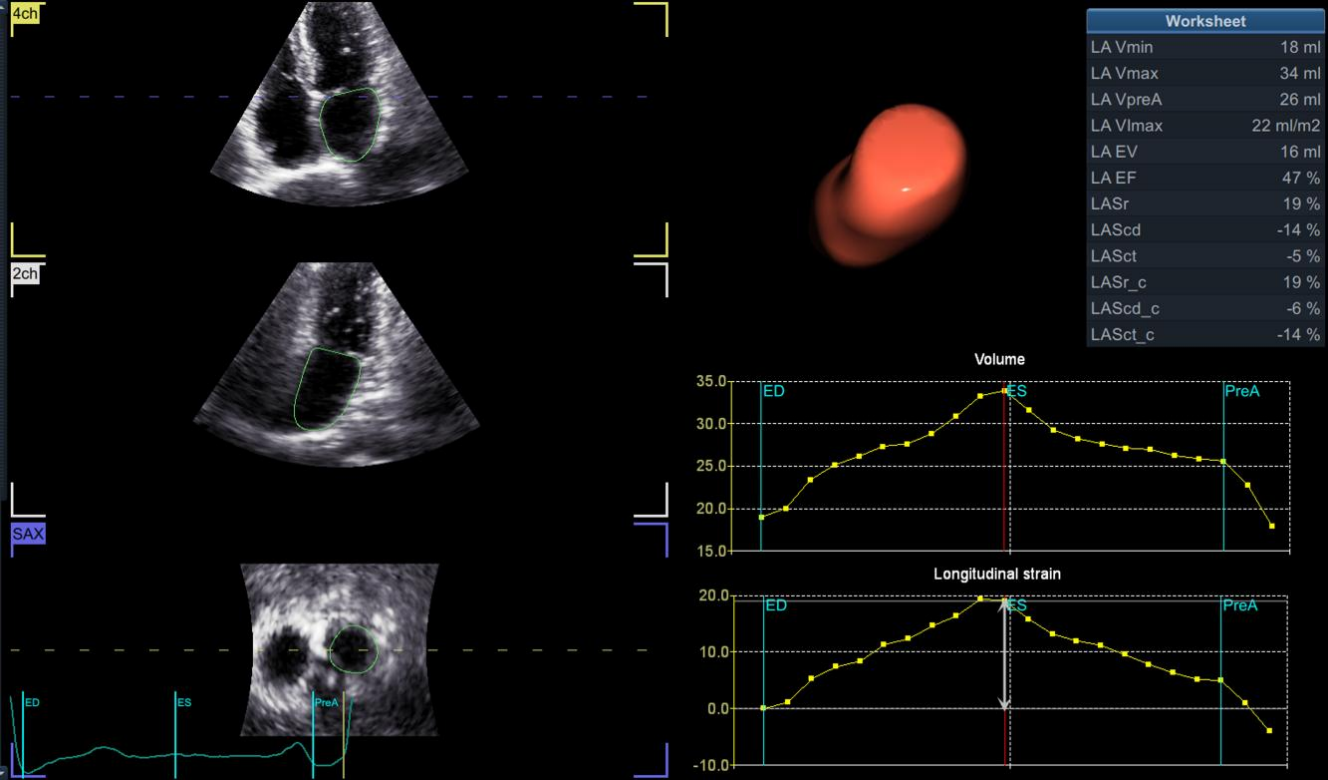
Three-dimensional speckle tracking echocardiographic quantification of left atrial volumes and function. (Left) Longitudinal and transverse visualization of three-dimensional echocardiographic left atrial volume. (Right) Left atrial volume–time and strain–time curves showing changes during the cardiac cycle. LA, left atrium; LAEF, total emptying fraction (total EV/Vmax); LAEV, total emptying volume (Vmax − Vmin); LAScd, longitudinal strain conduit phase; LAScd_c, circumferential strain conduit phase; LASct, longitudinal strain contraction phase; LASct_c, circumferential strain contraction phase; LASr, longitudinal strain reservoir phase; LASr_c, circumferential strain reservoir phase; LA-VImax, maximum volume index; LA-Vmax, maximum volume (at LV end-systole); LA-Vmin, minimum volume (at LV end-diastole); LA-VpreA, volume before atrial contraction (at LV early diastole). LA expansion index, LAEV/Vmin × 100.
